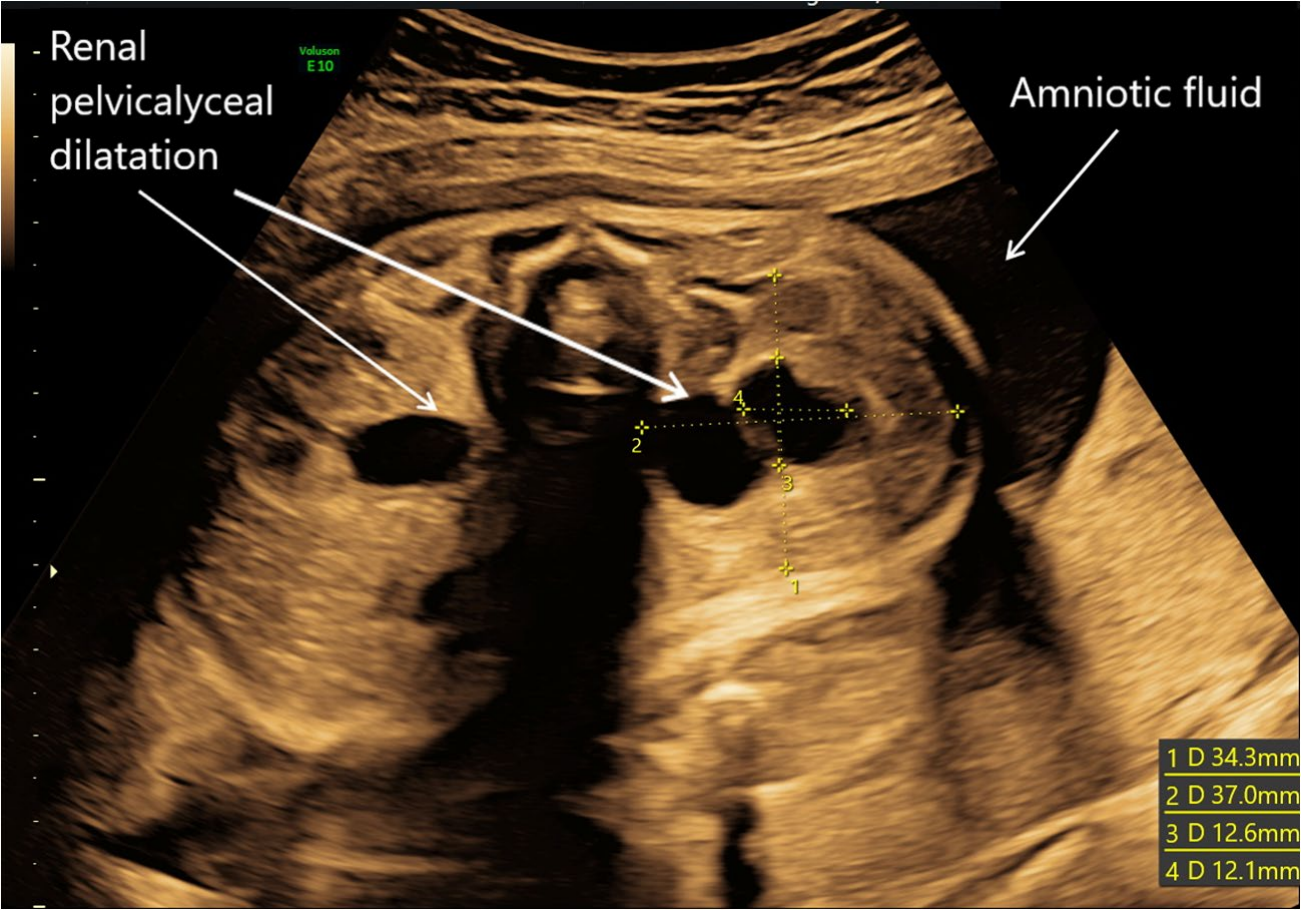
Antenatal ultrasound at 30/40 gestation showing bilateral renal pelvis and calyceal dilatation with normal amniotic fluid volume
